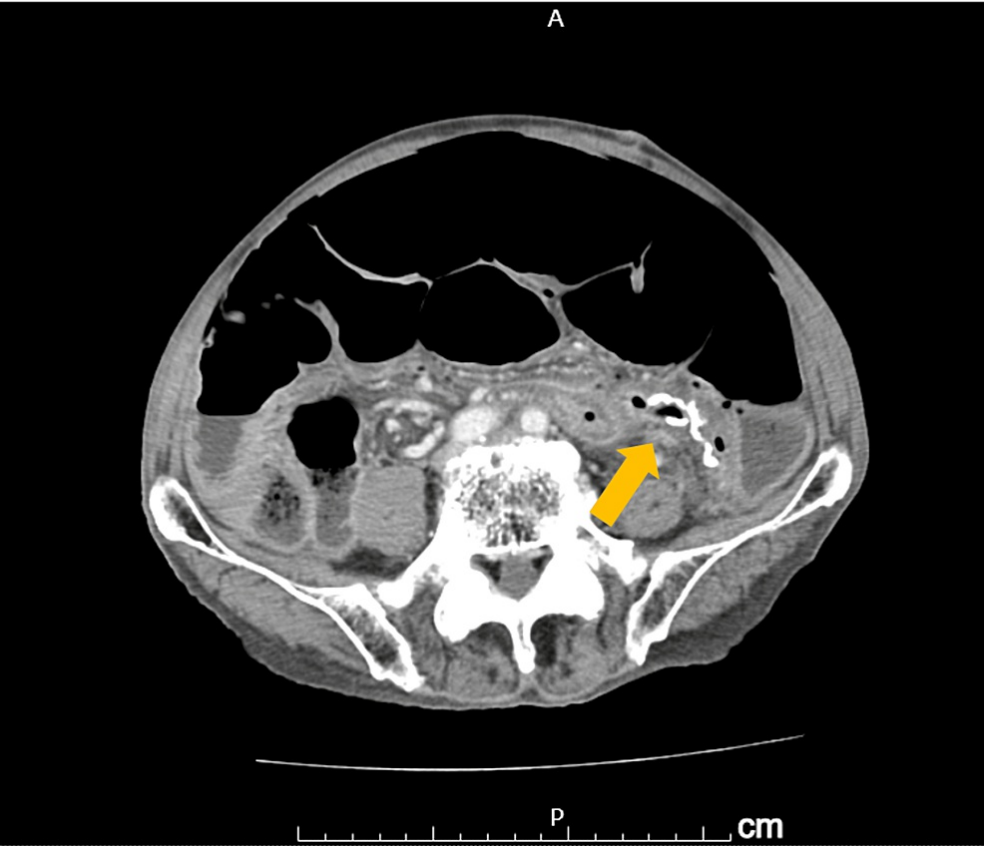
CT scan showing free air and ascites around the anastomotic site
